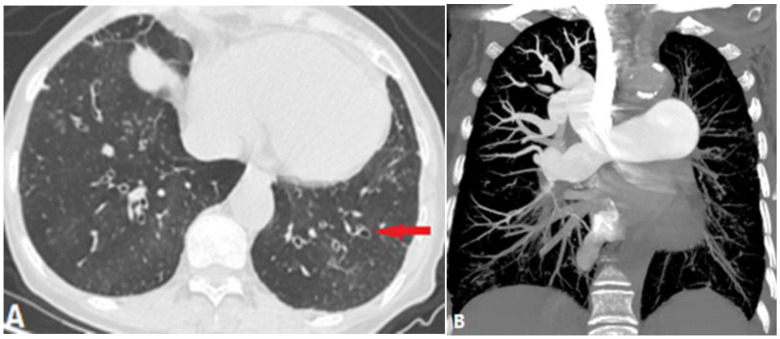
SMJS. A 71-year-old woman with Swyer–James–Macleod syndrome. (A): Lung window axial CT shows bronchiectasis and infections in the lower-right and -left lobes (arrow), and the hyperlucent right lung. (B): Coronal MIP of CTPA shows hypoplasia of the pulmonary vasculature of the left lung. CTPA—computed tomography pulmonary angiography; MIP—maximum-intensity projection.
